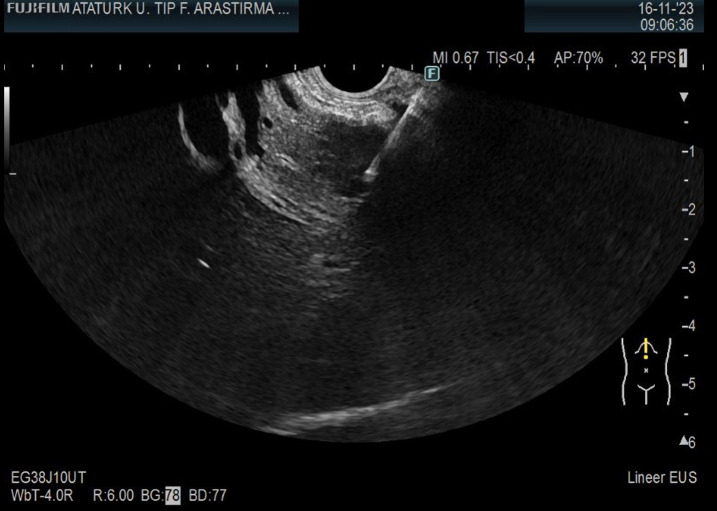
Endoscopic ultrasound-guided liver lesion biopsy performed in a patient where percutaneous biopsy could not be performed at the portal hilus.
